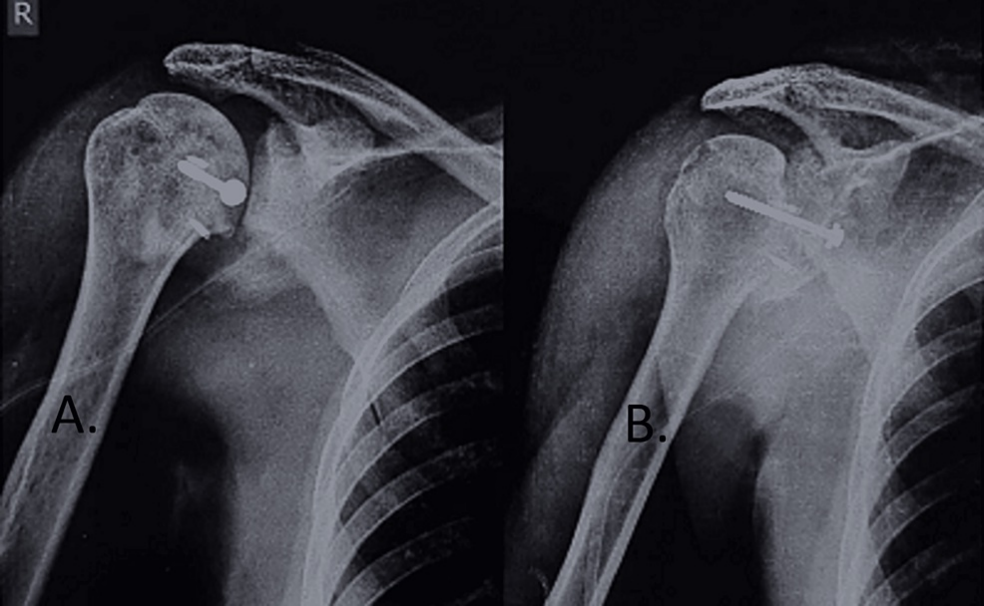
Postoperative X-ray of the patient with the bone chunk over the defect and implant in situ. (A) Anteroposterior view. (B) Lateral view of the proximal humerus.
